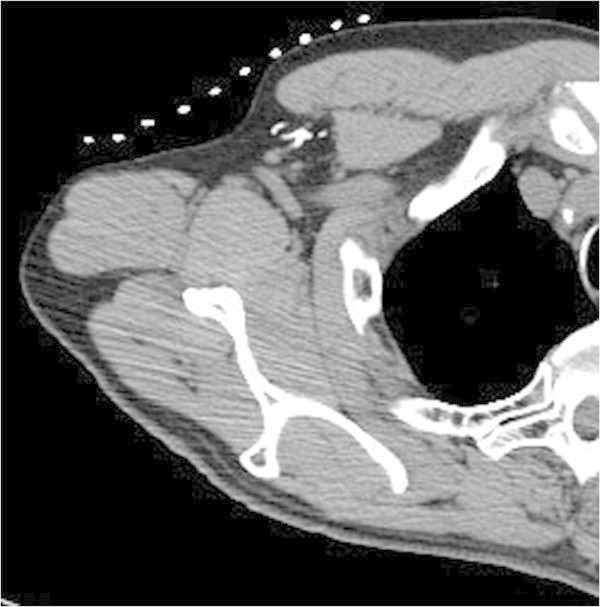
Computed tomographic lymphography provided clear visualization of the lymphatic vessels and sentinel lymph nodes in the axillary region.
